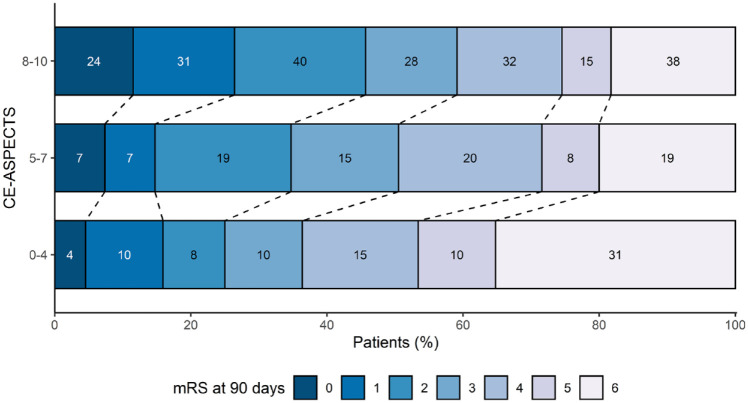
Modified Rankin Scale (mRS) distribution per contrast extravasation ASPECTS (CE-ASPECTS) category.
